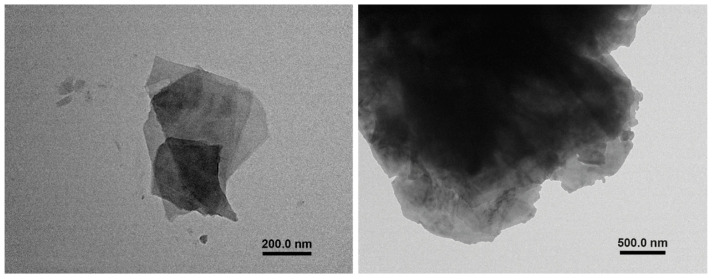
TEM images of HRCM powder after ultrasonication in isopropanol at two different length scales, showing the presence of superimposed high-ratio flakes with few layers of graphene sheets at the micro-sized length scale.
